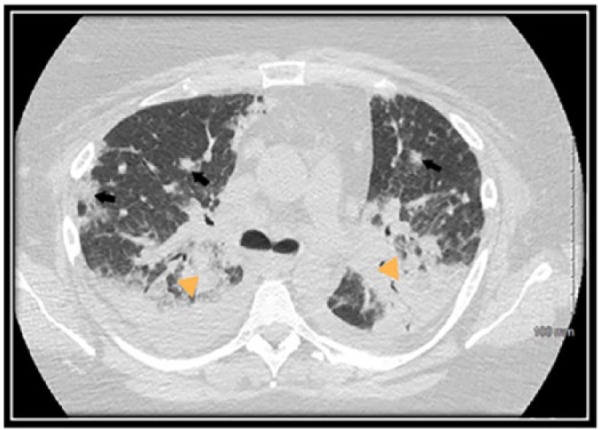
Chest computed tomography scan on admission demonstrating diffuse nodular opacities with spiculated peripheral ground opacities (arrows) and bilateral lower lobe consolidation (arrow heads).
